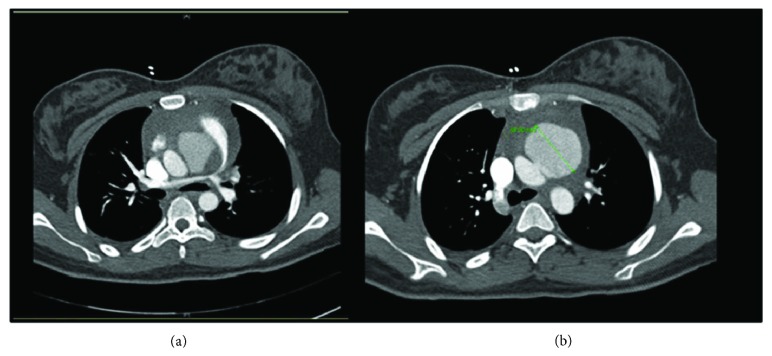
CT scans identifying pseudoaneurysm. Pseudoaneurysm compressing on the pulmonary trunk and right main stem (a) and 4.8 cm aortic pseudoaneurysm (b).
